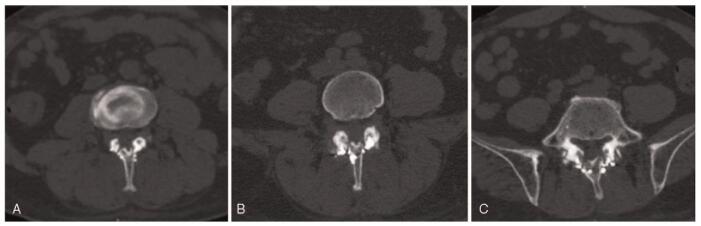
Postoperative computed tomography scan showed that the resected lamina was replanted in situ. (A) L3–L4 level, (B) L4–L5 level, and (C) L5–S1 level.
